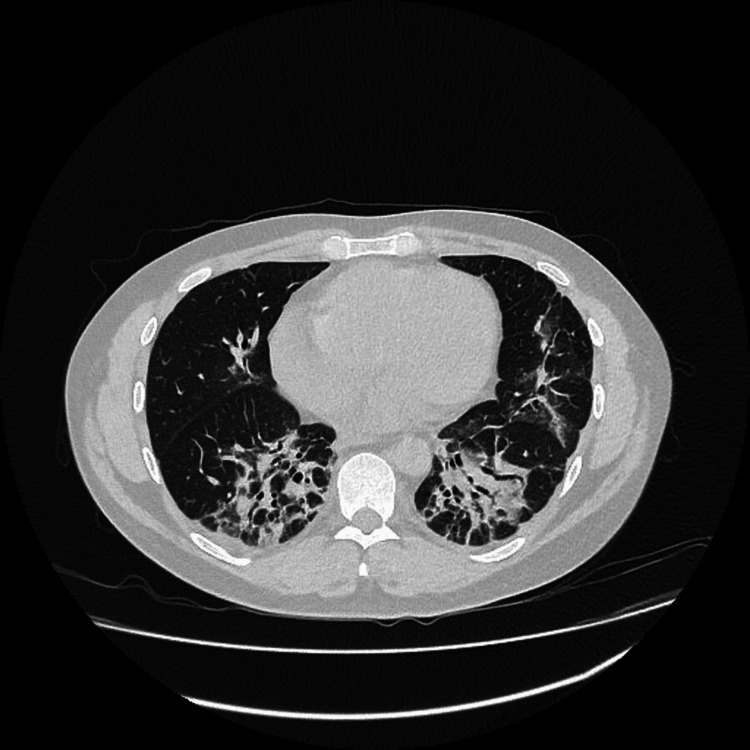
A high-resolution CT scan shows organizing pneumonia pattern and appears to be resolving
